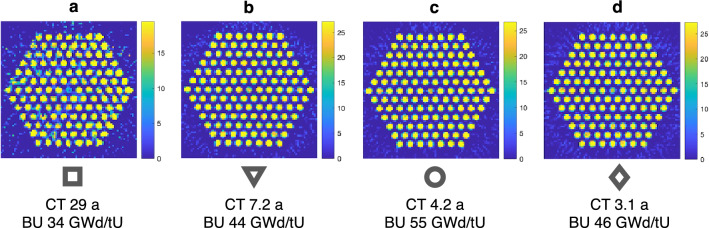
Reconstructed gamma activity images in the 1200–1300 keV window for the same four VVER-440 assemblies (a–d) as in Fig. 6b. Symbols used in Fig. 6b to denote the assemblies are shown below each image here, along with the CT and BU information.
